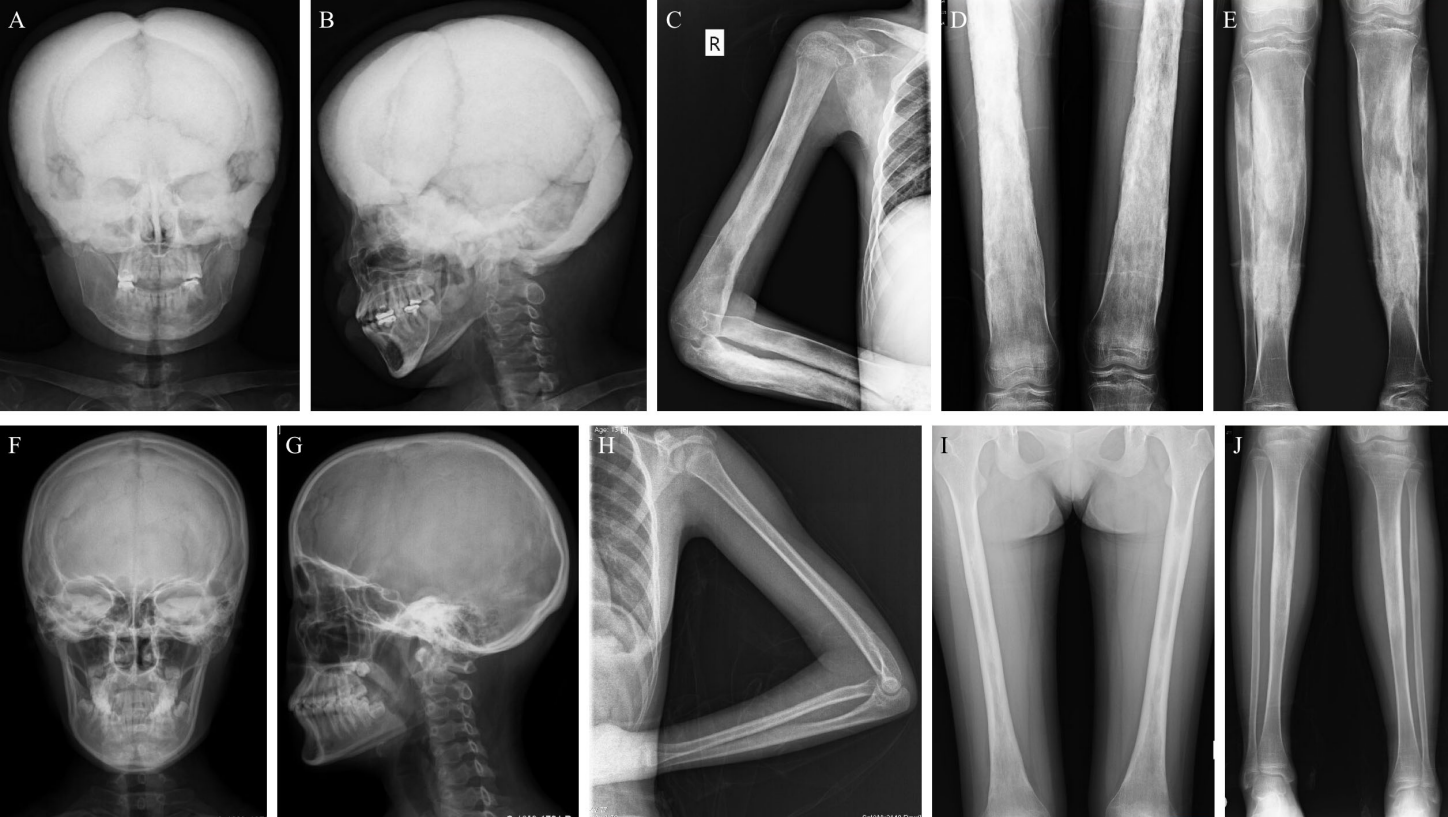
Typical X-rays of patients with CED. (A–E) are relatively severe radiographs of an 18 years old female with CED, and (F–J) show mild changes in radiographs of patients with CED (F–I: female/13 years old; J: female/18 years old). (A, B) The skull and skull base have severe sclerosis and thickening on the anteroposterior and lateral skull radiographs. (C–E) Obvious cortical thickening diaphysis and narrowed medullary canal in the humerus, radius, ulna, femur, tibia, and fibula. (F, G) The skull and skull base have no obvious thickening or sclerosis. (H) Slight cortical thickening diaphysis and narrowed medullary canal in the ulna but not the humerus or radius. (I, J) Mild cortical thickening diaphysis and narrowed medullary canal in the femur and tibia. CED, Camurati-Engelmann disease.
